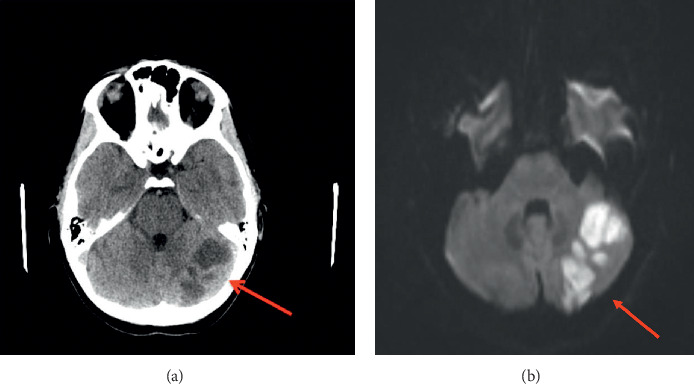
Noncontrast CT of the head (a) and brain MRI-DWI sequence (b) showing several well-circumscribed near fluid attenuation regions in the left cerebellum, the largest of which measures 3.5 cm transverse diameter. This is suggestive of left cerebellar ischemic stroke. There is no acute hemorrhage, midline shift, or hydrocephalus.
